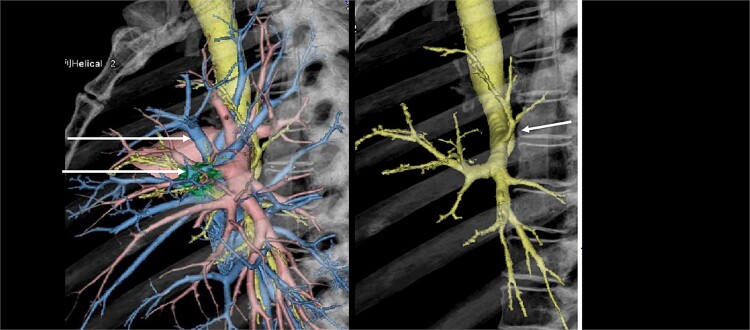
Preoperative three-dimensional contrast-enhanced computed tomographies (3DCT) showed a displaced anomalous B1^+2^ bronchus arising from left main bronchus, which ascends behind the main pulmonary artery.
